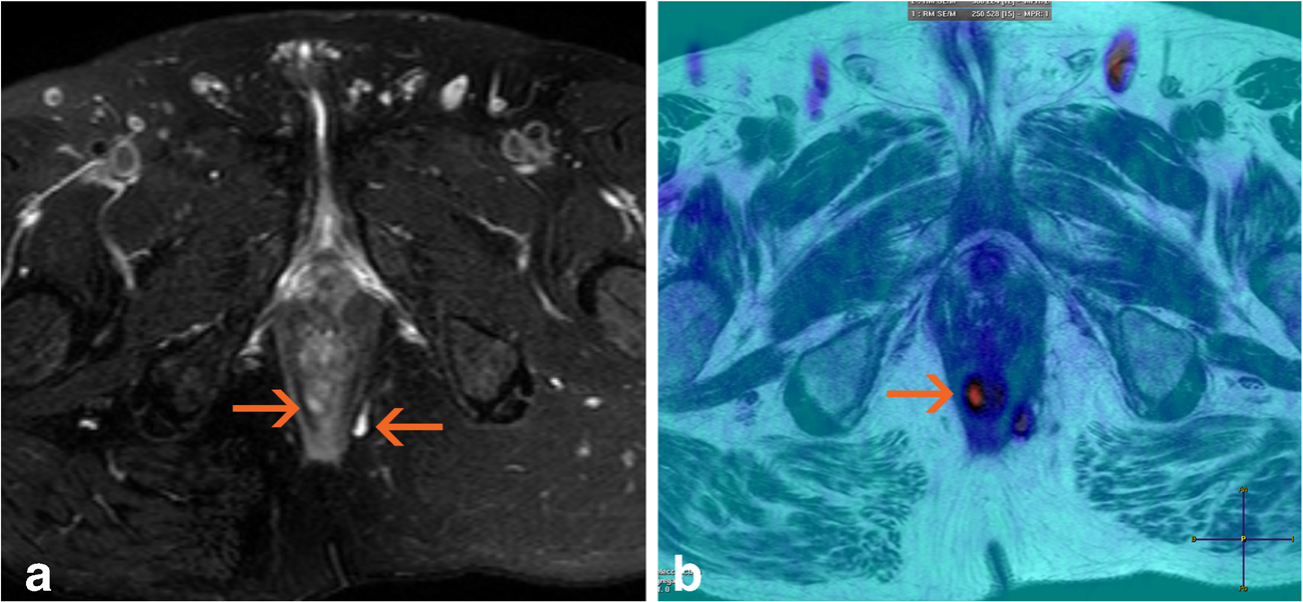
Oblique-transverse T2-weighted image (a) and merged view of a colour-coded map derived from an 800 s/mm^2^ b factor and a T2-weighted image (b). Right intersphinteric path (arrow) is clearly more conspicuous in image B
